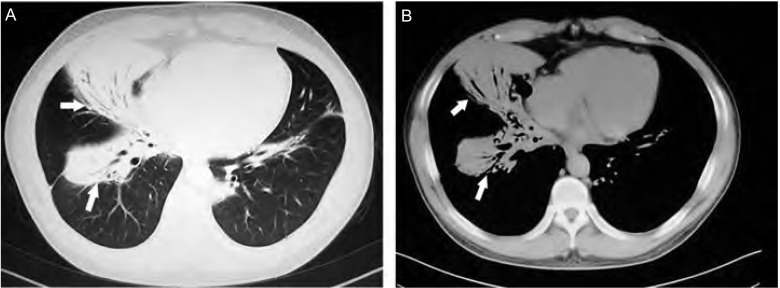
胸部CT显示右肺中叶大片实变影伴支气管气相征（箭头所指）。A：肺窗；B：纵隔窗 Extensive consolidation with air bronchogram in the middle lobe of right lung on CT scans (Arrow). A: pulmonary window; B: mediastinum window
